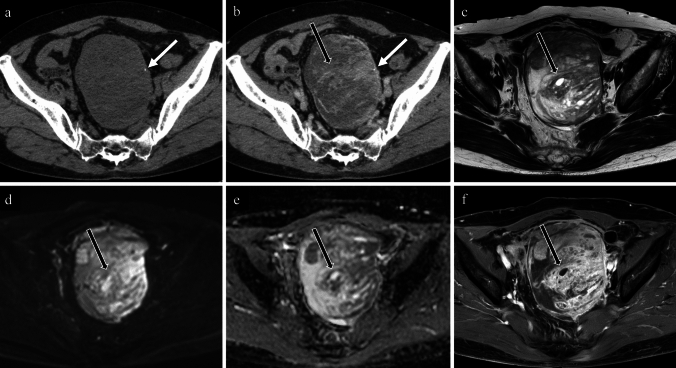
67-year-old woman with a clear cell carcinoma of the left ovary. a non-contrast CT; b contrast-enhanced CT; c axial T2-weighted image; d axial diffusion-weighted image; e axial apparent diffusion coefficient map; f axial contrast-enhanced T1-weighted image. Non-contrast CT reveals a single punctate calcification in the left wall of the cystic tumour (a; white arrow). On contrast-enhanced CT, the papillary solid tissues are well-enhanced (b; black arrows), making the calcification less prominent (b; white arrow). On T2-weighted imaging, a unilocular cystic lesion with tall, papillary solid tissues is observed, and the solid tissues show intermediate signal intensities and contain small internal cystic components (c; black arrow). Strong diffusion restriction and significant enhancement are observed in the solid tissues (d–f; black arrows). Pathological examination confirmed a clear cell carcinoma associated with endometriosis
